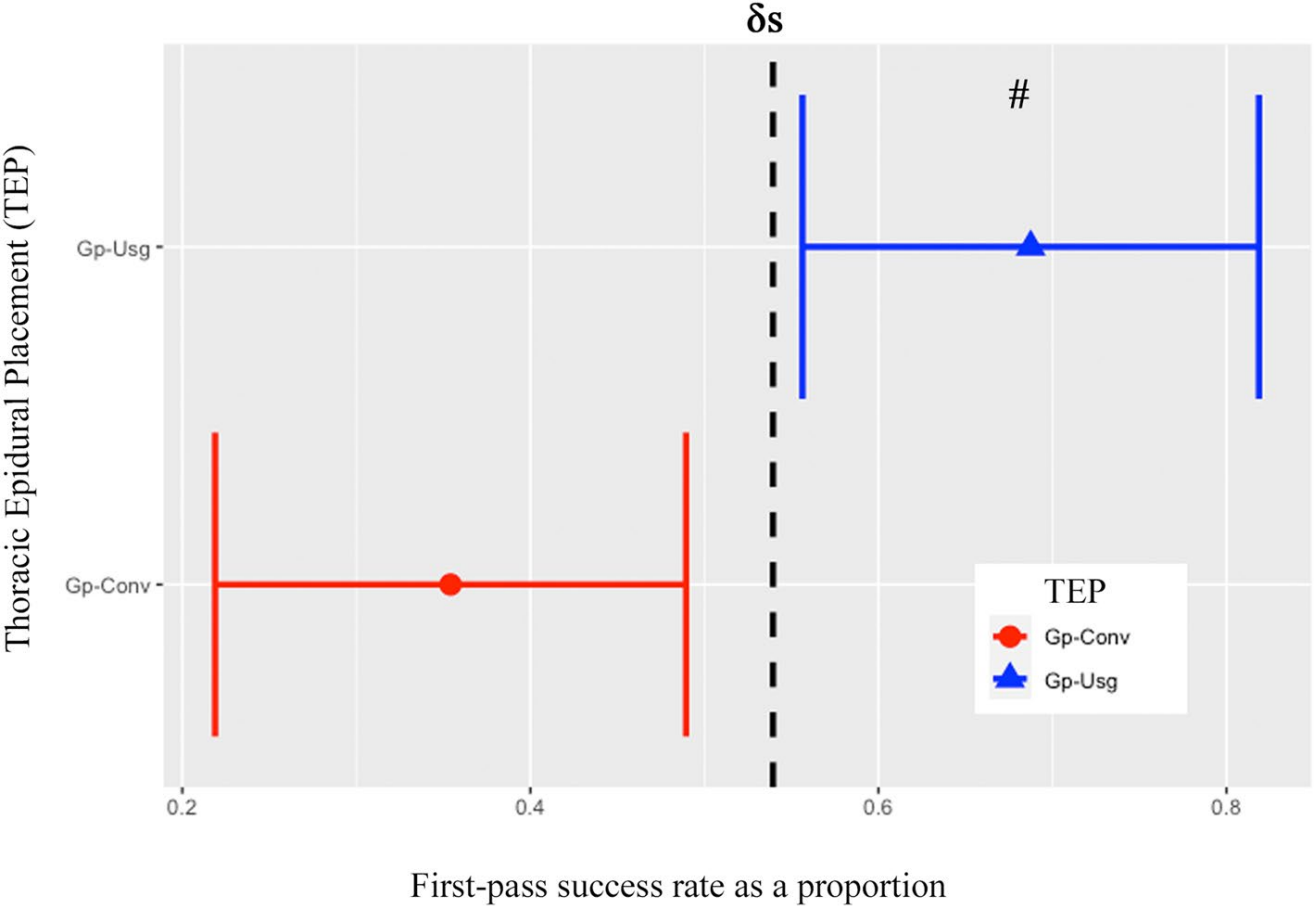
The first-pass success rate of thoracic epidural placement in the study groups. Data are presented as a proportion with its 95% CI (confidence interval). The Black dashed line represents the pre-specified superiority margin (δs = 0.05). Note the lower bound of the 95%CI for the first-pass success rate of Gp-Usg (real-time ultrasound-guided group) does not overlap the upper bound of the 95%CI of Gp-Conv (conventional anatomic landmark group) and it is beyond the pre-specified δs of 5% confirming clinical superiority of Gp-Usg. # represents inter-group difference *p* = 0.002, and TEP, thoracic epidural placement
